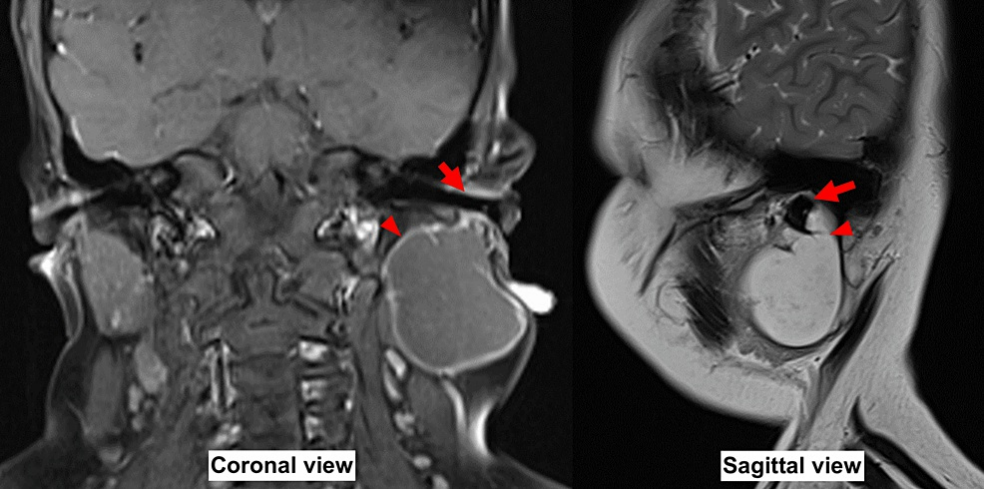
MRI of the neck revealing a well-defined lobulated intra-glandular cystic lesion (arrowhead) within the left parotid gland measuring 4x4cm near the external auditory canal (arrow). First image in T1 coronal view showing a hypointense lesion. Second image in T2 sagittal view showing a hyperintense lesion.
